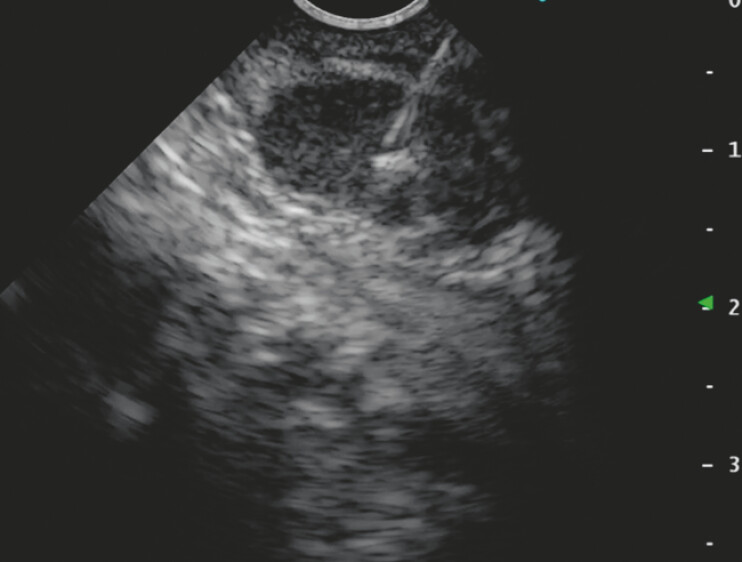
Endoscopic ultrasound-guided fine-needle biopsy was performed using a 22-gauge needle.
